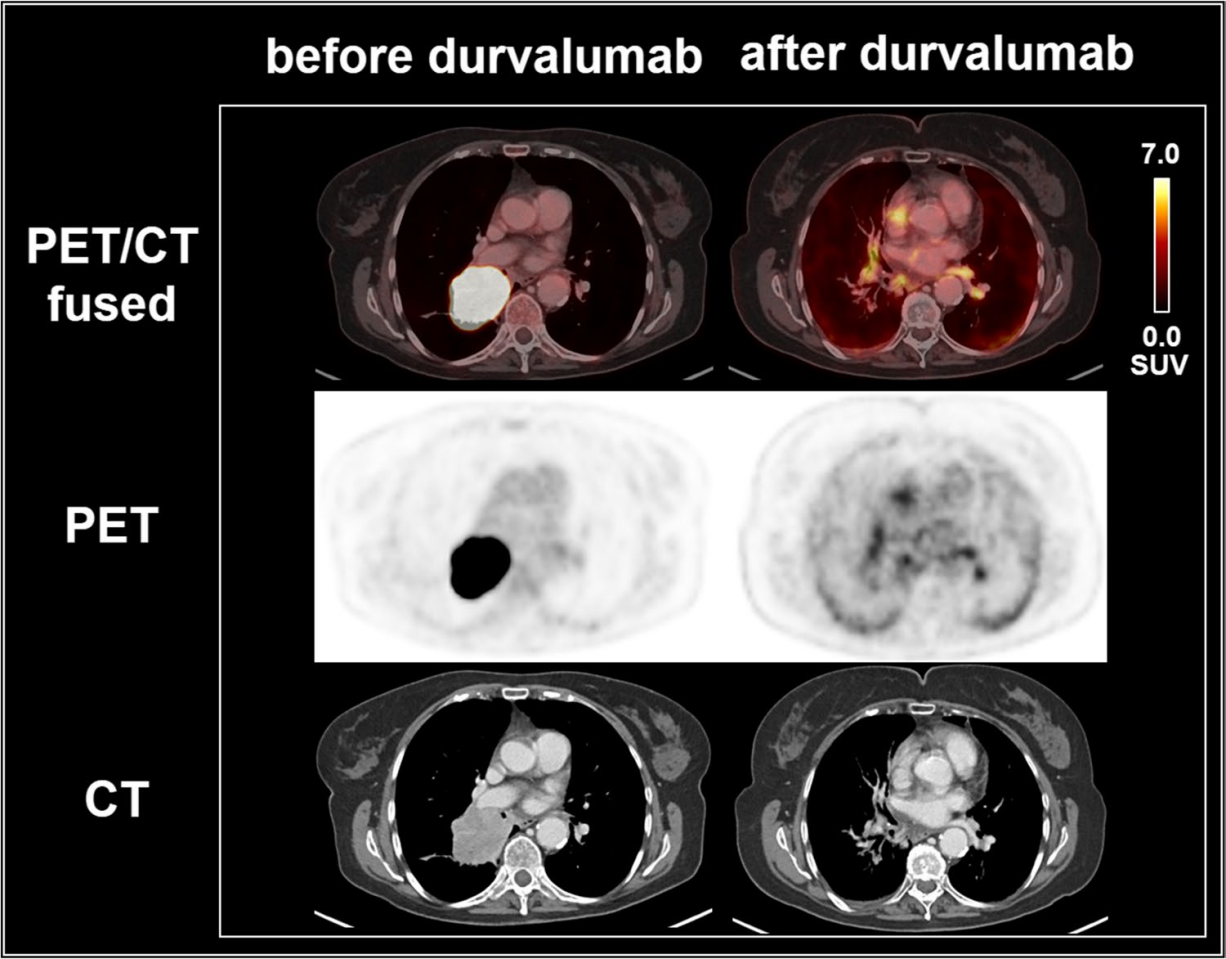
[^18^F]FDG PET/CT scans of a 71-year old woman with stage IIIB NSCLC of the right upper lobe, cT3 cN2 cM0, adenocarcinoma, ALK-negative, PD-L1: 40%, former smoker (30 py), undergoing CRT with 63.6 Gy, Cisplatin/Vinorelbine, and durvalumab maintenance treatment, who developed findings suggestive of an immunotherapy-related sarcoid-like reaction. After durvalumab administration, mediastinal and hilar lymph nodes were slightly enlarged and showed an increased uptake on [^18^F]FDG PET/CT (SUV_max_ 9.0). Follow-up imaging 3.5 months later revealed a decreasing size and a strongly decreasing, now normalized glucose metabolism of the thoracic lymph nodes, consistent with a prior immunotherapy-related sarcoid-like reaction. The patient had a PFS of 11 months and was still alive at the last follow-up after 34 months
